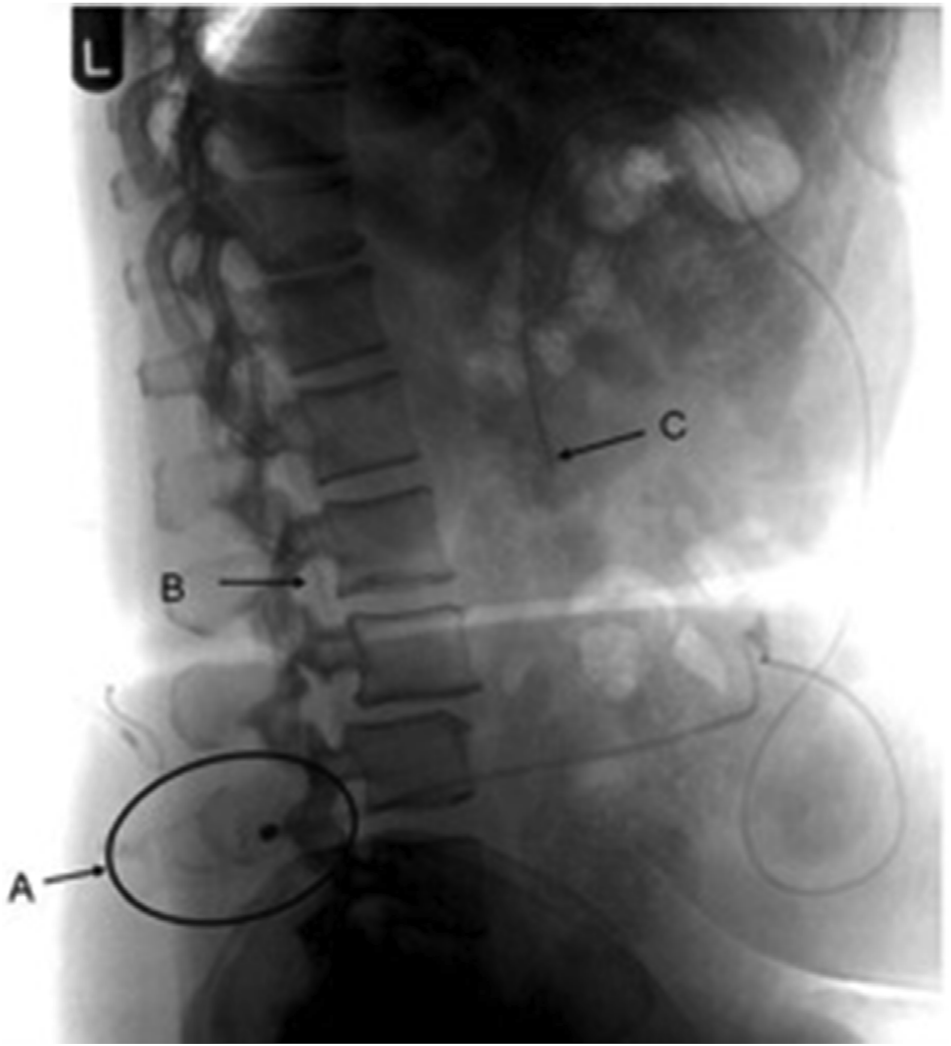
Lateral abdominal radiograph confirming an LP shunt with its proximal catheter within the spinal canal (B), a valve (A), and the distal catheter tip (C) lying intraperitoneally. Figure adapted from Toma et al., 2010 [2].
